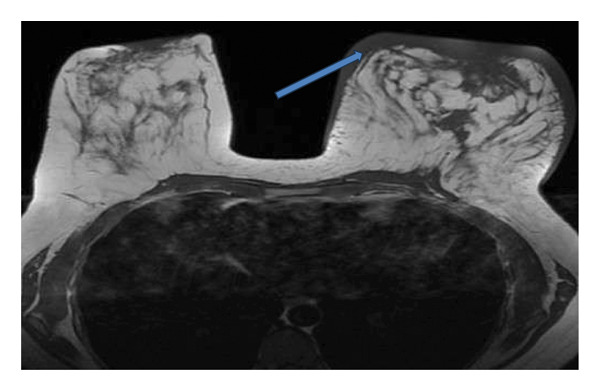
The same patient as in Figure 17. The MRI T2‐weighted sequence shows breast asymmetry, with the left breast larger in size and associated with diffuse thickening of the skin (18).
